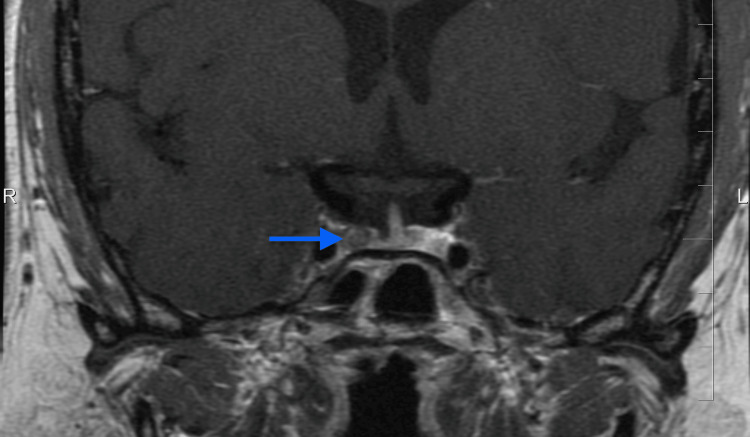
MRI demonstrating a second recurrence of pituitary adenoma (blue arrow)
